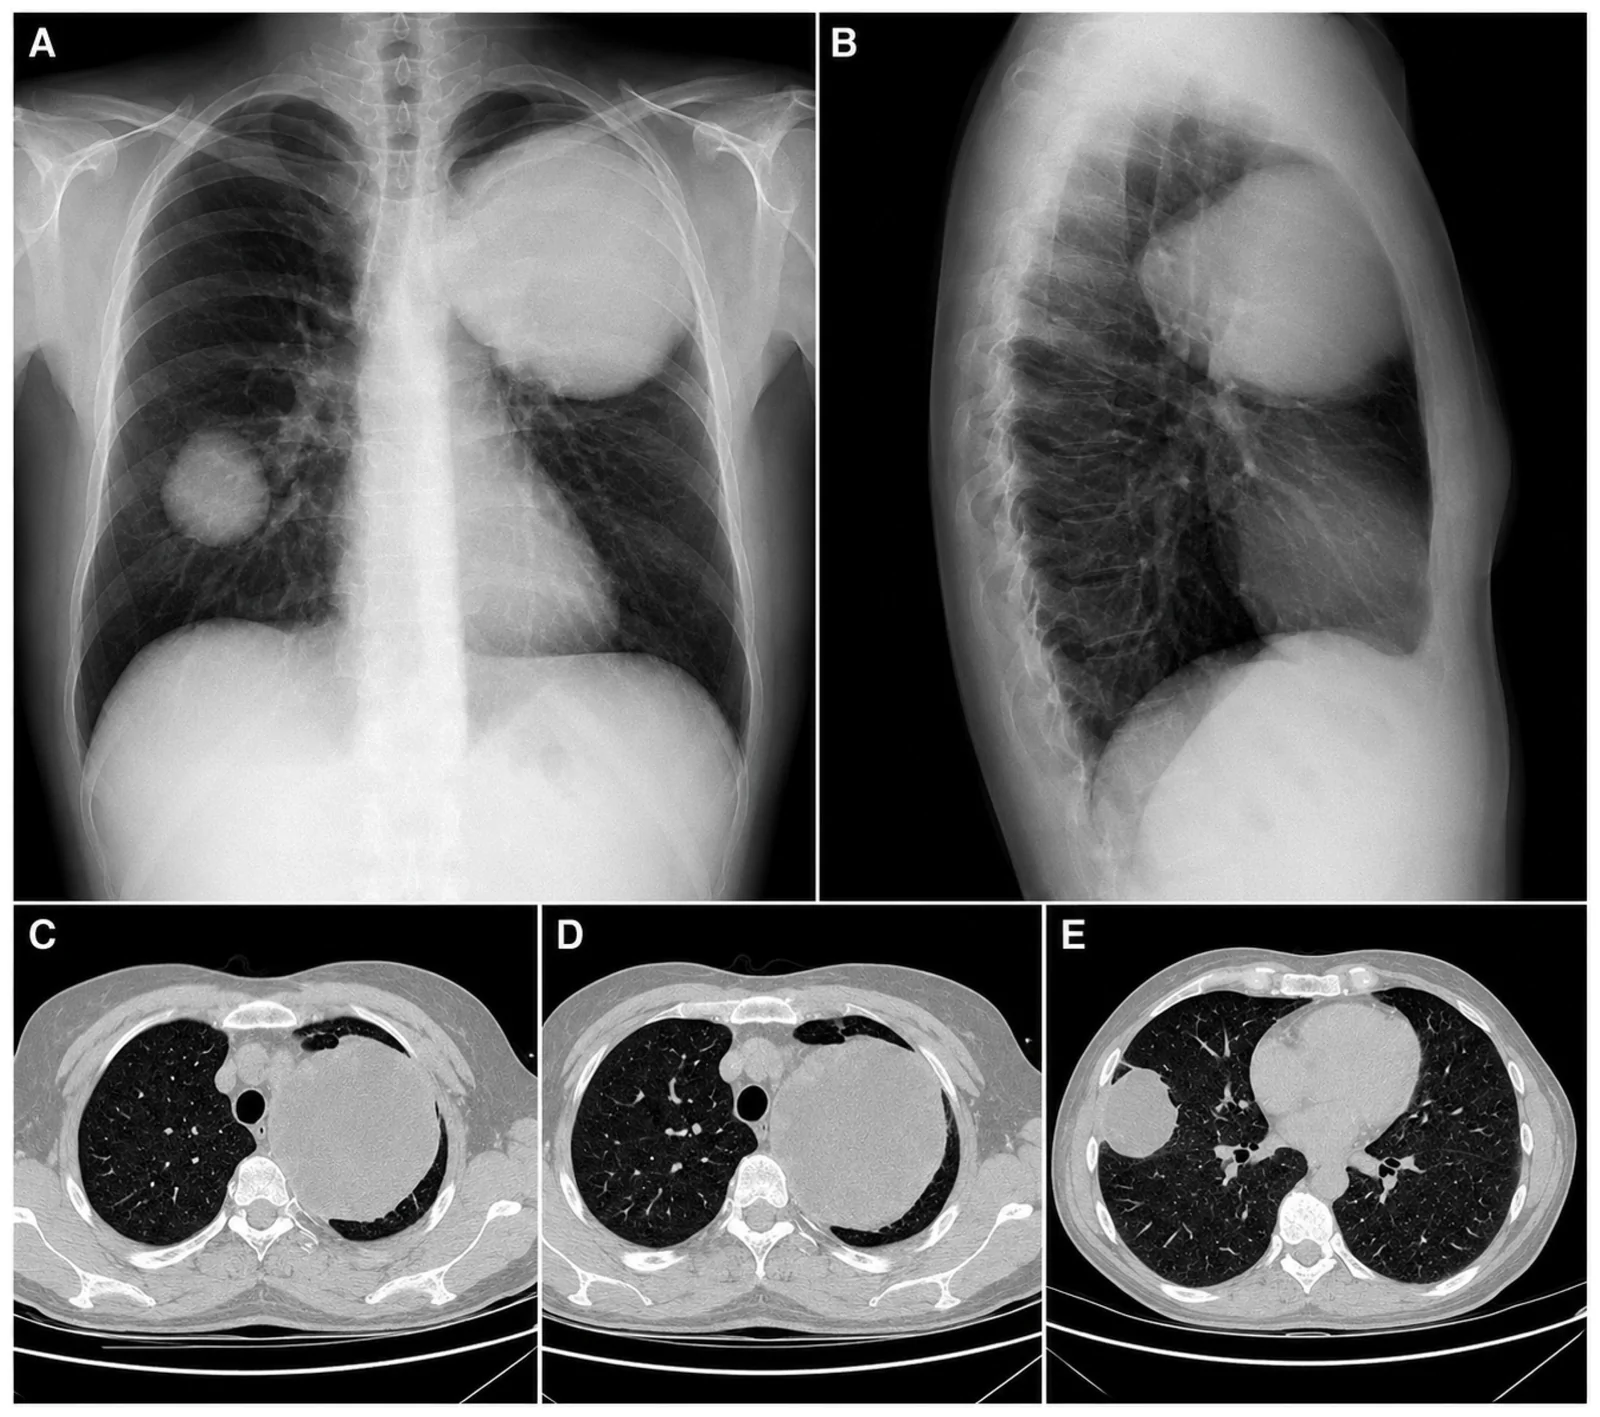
Chest radiography and computed tomography findings at initial presentation. (A). Posteroanterior chest radiograph showing two well-defined rounded pulmonary masses, including a large lesion projected over the left upper lung field and a smaller lesion projected over the right middle lung field. (B). Lateral chest radiograph confirming the posterior/apical projection of the larger thoracic mass. (C,D). Axial chest CT images showing a large solid mass in the left upper lobe/apical region, with broad pleural and mediastinal contact. (E). Axial chest CT image showing a second well-defined solid lesion in the right middle lobe, with pleural contact and lateral pleural involvement.
